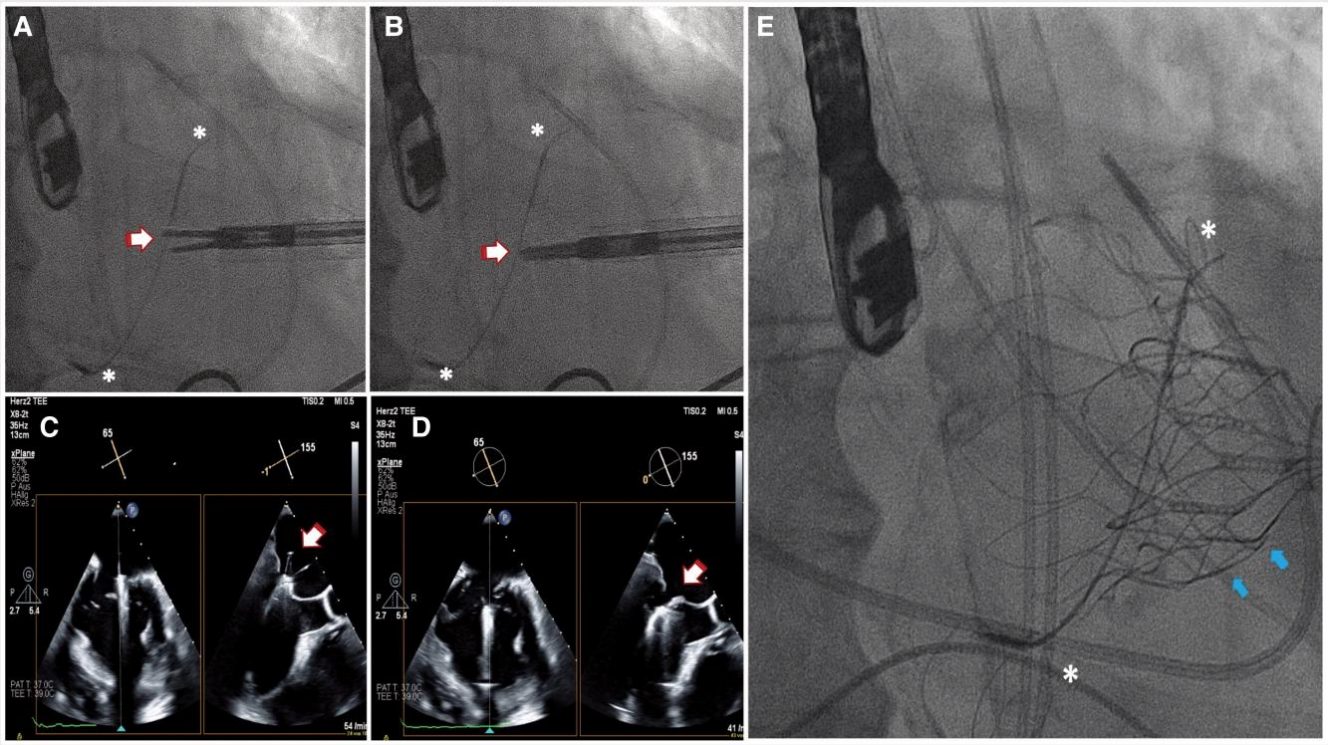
Simultaneous intraprocedural TEE and fluoroscopic procedural images. (*A–B*) Fluoroscopic images show the Carillon device (asterisks), the sheath, and the endoscopic scissor just before and after the laceration of the anterior mitral leaflet (arrows). (*C–D*) 2D TEE multi-plane imaging (X-plane) guidance shows the simultaneous bi-commissural and LVOT views before and after the MitraCut procedure with scissor blades (indicated by the arrow). (*E*) Fluoroscopic views of the Carillon® Mitral Contour System™ device implanted in the coronary sinus (asterisks) and TMVR (arrows) with a SP 33M Tendyne system (Abbott Structural Heart, Santa Clara, CA, USA). 2D TEE, two-dimensional transoesophageal echocardiography; TMVR, transcatheter mitral valve replacement; SP, standard profile; LVOT, left ventricular outflow tract.
